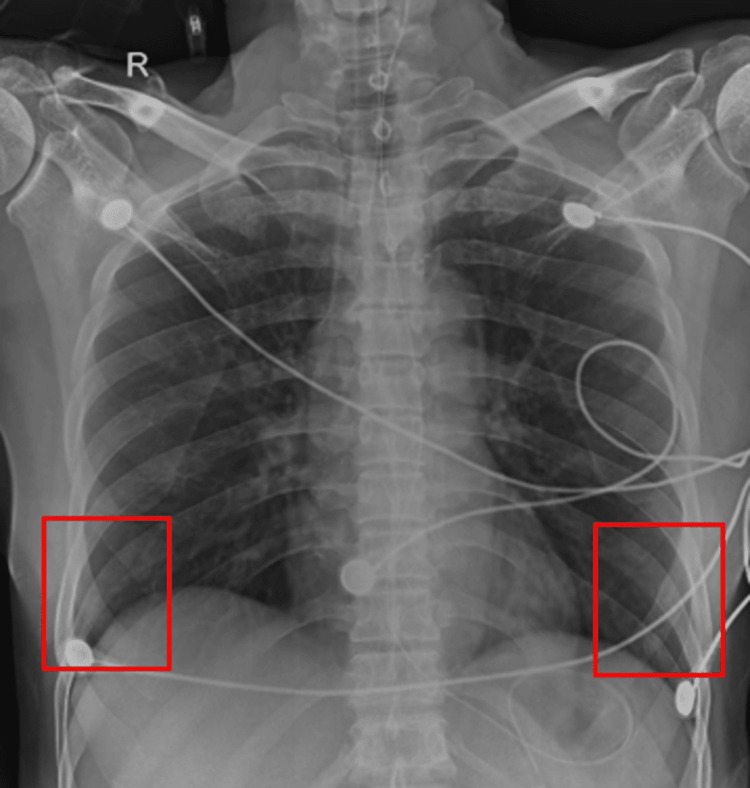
AP view of the chest X-ray taken on day 7 showing translucency AP: anteroposterior
